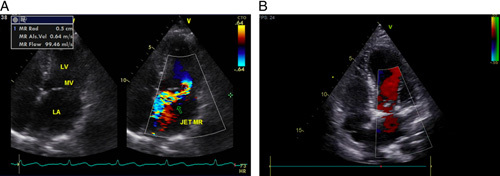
Patient 1 6 months evaluation and post-MV repair echocardiography. (A) Echocardiography evaluation revealed severe MR; (B) echocardiography post-MV repair.
